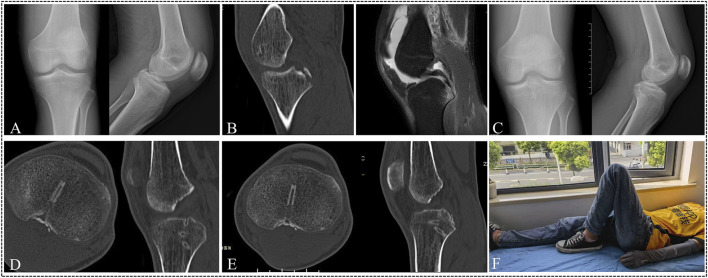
A 26-year-old male suffered a displaced PCL tibial avulsion fracture of the left knee as a result of motorcycle traffic. (A–C) The X-ray, CT, and MRI images demonstrated the presence of a PCL tibial avulsion fracture. (D) The X-ray images showed fracture fragment reduction well at 2 weeks after operation. (E) The CT images revealed that there was no evidence of fracture displacement at 6 weeks after the operation. (F) At 10 weeks, the CT image demonstrated evidence of fracture union. G At 12 weeks, the patient was satisfied with the surgical outcome and returned to previous work.
